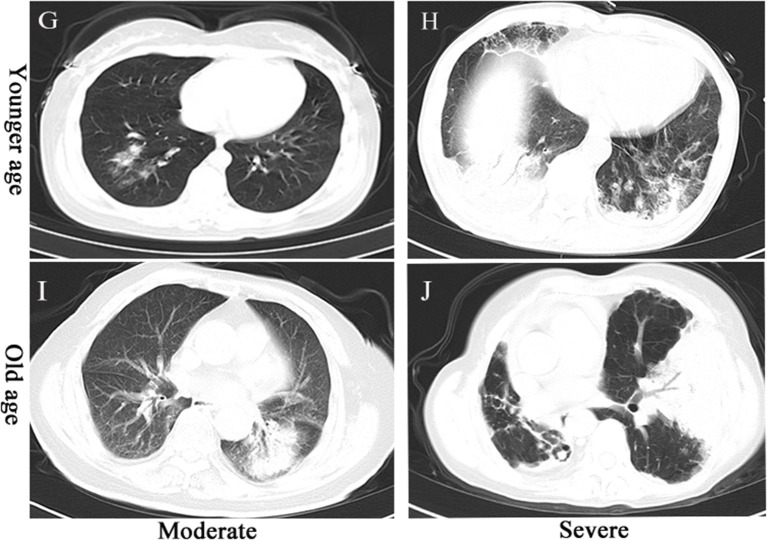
CT imaging of SN-CAP patients. (G) Moderate-severity pneumonia in a 49-year-old woman presenting as patchy exudative shadows in the right upper lobe. (H) Severe pneumonia in a 50-year-old man presenting as large exudative shadows in both lungs. (I) Moderate-severity pneumonia in a 90-year-old man presenting as exudative shadows in the left lower lobe. (J) Severe pneumonia in an 80-year-old woman presenting as large exudative shadows in both lungs.
